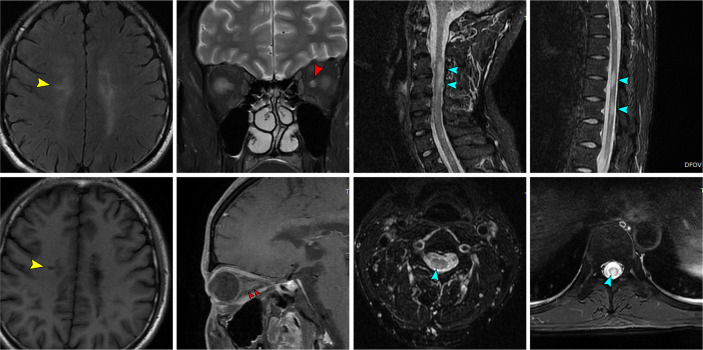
The patient’s MR images which mimicking MS.
